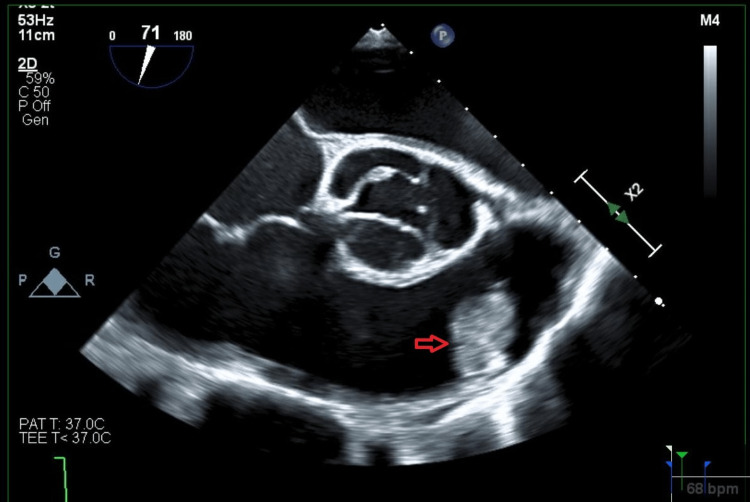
Mid-esophageal right ventricle inflow and outflow view on transesophageal echocardiogram. Suspected myxoma (red arrow) seen on the pulmonic valve leaflet.
